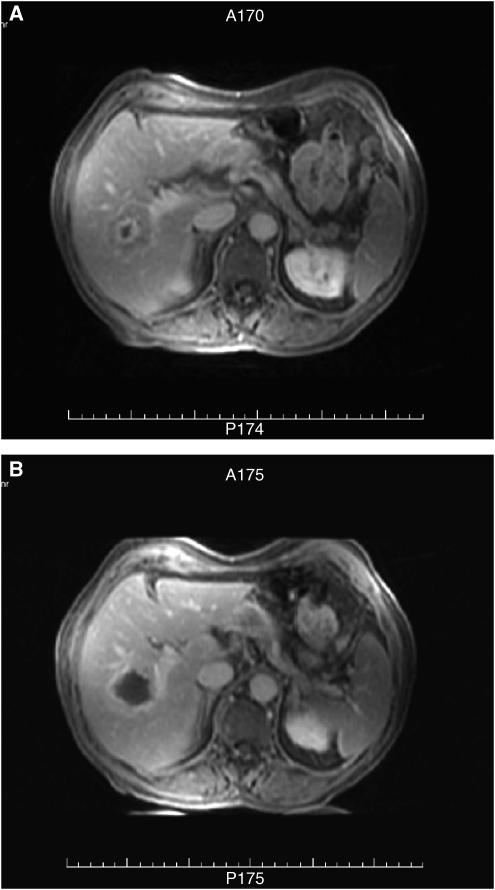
Axial FAME series MRI images, 1 min post IV gadolinium contrast. (A) Before HIFU with a right hepatic metastasis within segment VIII showing central necrosis, and (B) 12 days after HIFU, a larger zone consistent with coagulation necrosis within the metastasis.
